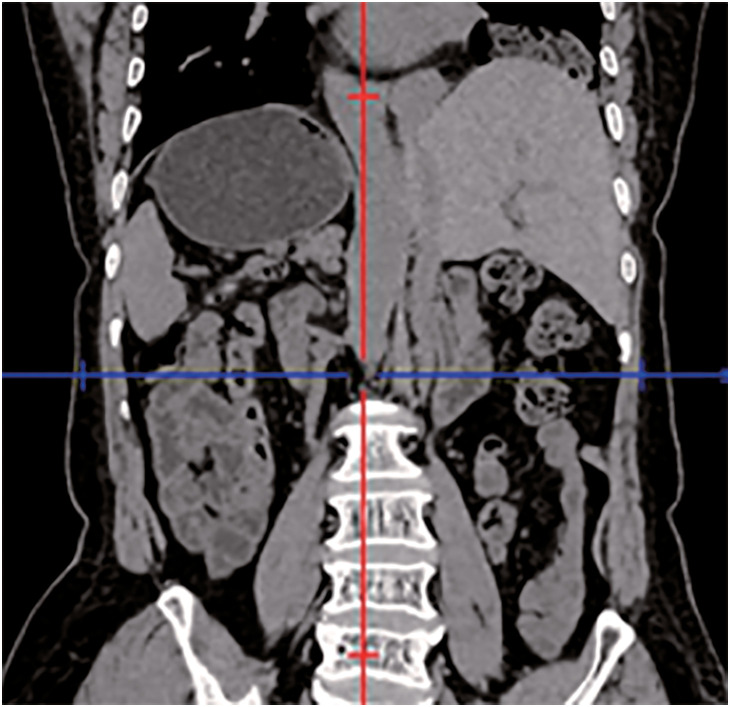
CT of the abdomen (coronal plane). The abdominal CT revealed complete situs inversus totalis, characterized by a mirror-image reversal of organs along the body’s left–right axis: a left-sided liver, right-sided spleen, a predominantly right-sided gastric bubble, and so on. CT: computed tomography
